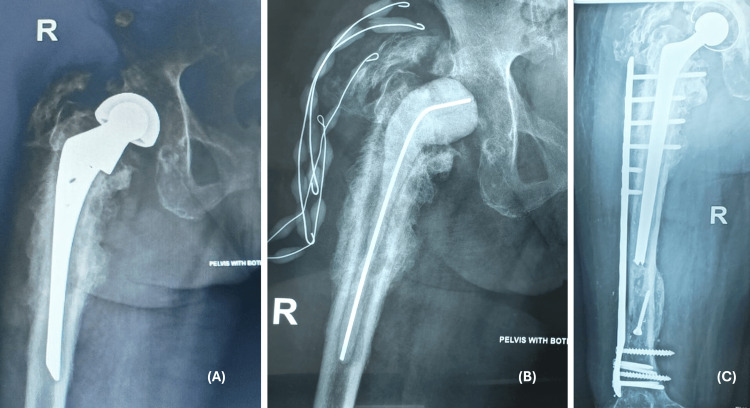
Radiology series of a 74-year-old female with heterotrophic ossification (A) Preoperative X-ray with Grade 2 acetabular erosion, (B) X-ray after first-stage surgery with an antibiotic cement spacer and beads, and (C) X-ray at final follow-up with total hip replacement (THR) prosthesis, also showing the plate and screws, which were used to manage the intraoperative fracture.
